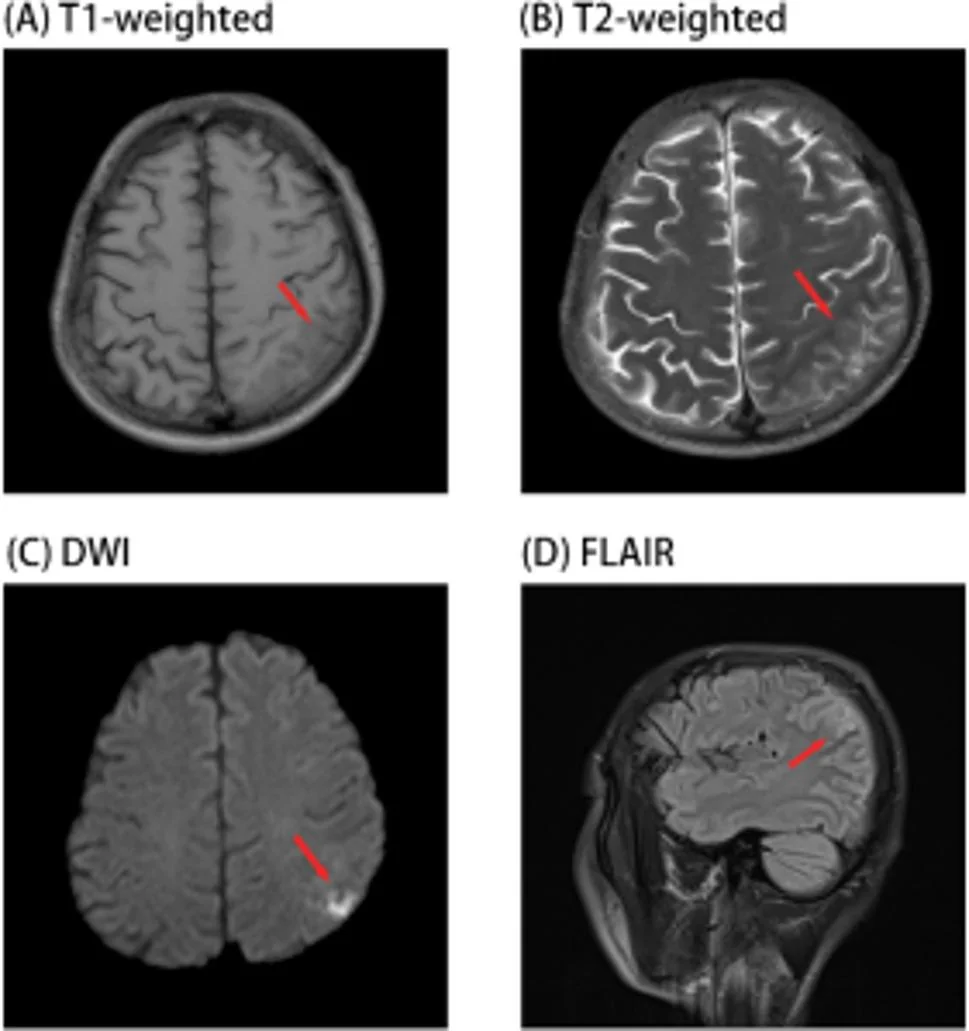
Brain Magnetic Resonance Imaging (MRI) Findings. Brain MRI showed a left parietal lobe hypointense lesion (arrow) on T1-weighted sequence (A) and hyperintense lesions (arrow) on T2-weighted (B), diffusion-weighted imaging (DWI) (C), and fluid-attenuated inversion recovery (FLAIR) (D) sequences before treatment
